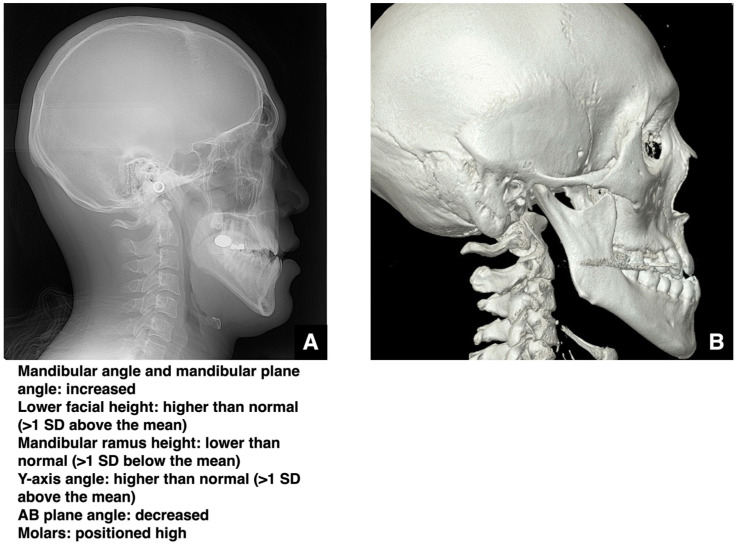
Analysis of skeletal mandibular prognathism with open bite. (A) Lateral cephalometric radiograph and summary of polygonal analysis of a patient with skeletal mandibular prognathism and open bite. (B) Reconstructed MDCT image (slice thickness, 0.5 mm) of a patient with skeletal mandibular prognathism and open bite. Permission has been obtained to publish all figures.
